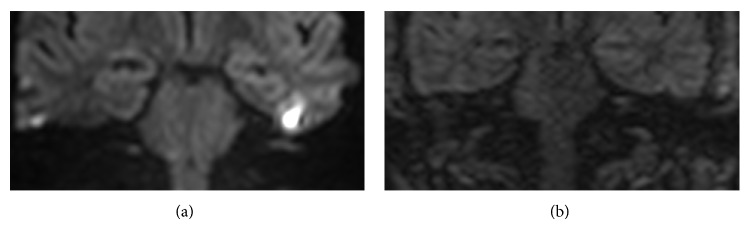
Images of left middle ear cavity in patient with chronic effusive otitis media and suspicion of recurrent cholesteatoma: both coronal SSh SE-EPI DWI (a) and coronal MSh TSE DWI (b) were negatives for cholesteatoma.
